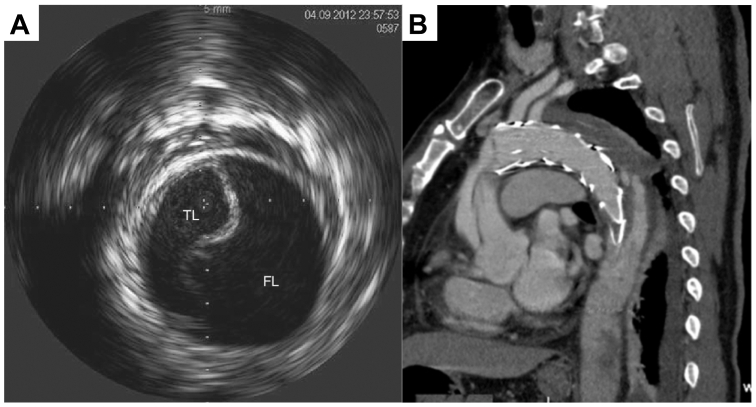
(A) Example of intravascular ultrasound (IVUS) confirmation of retrograde type A dissection (RTAD) in aortic arch. (B) CTA of retrograde type A dissection after thoracic endovascular aortic repair (TEVAR) repair. (Reproduced from Wendt D, Thielmann M, Melzer A, et al. The past, present and future of minimally invasive therapy in endovascular interventions: a review and speculative outlook. Minim Invasive Ther Allied Technol 2013;22:242-253. Ma T, Dong ZH, Fu WG, et al. Incidence and risk factors for retrograde type A dissection and stent graft-induced new entry after thoracic endovascular aortic repair. J Vasc Surg 2018;67:1026-1033.e2.).
